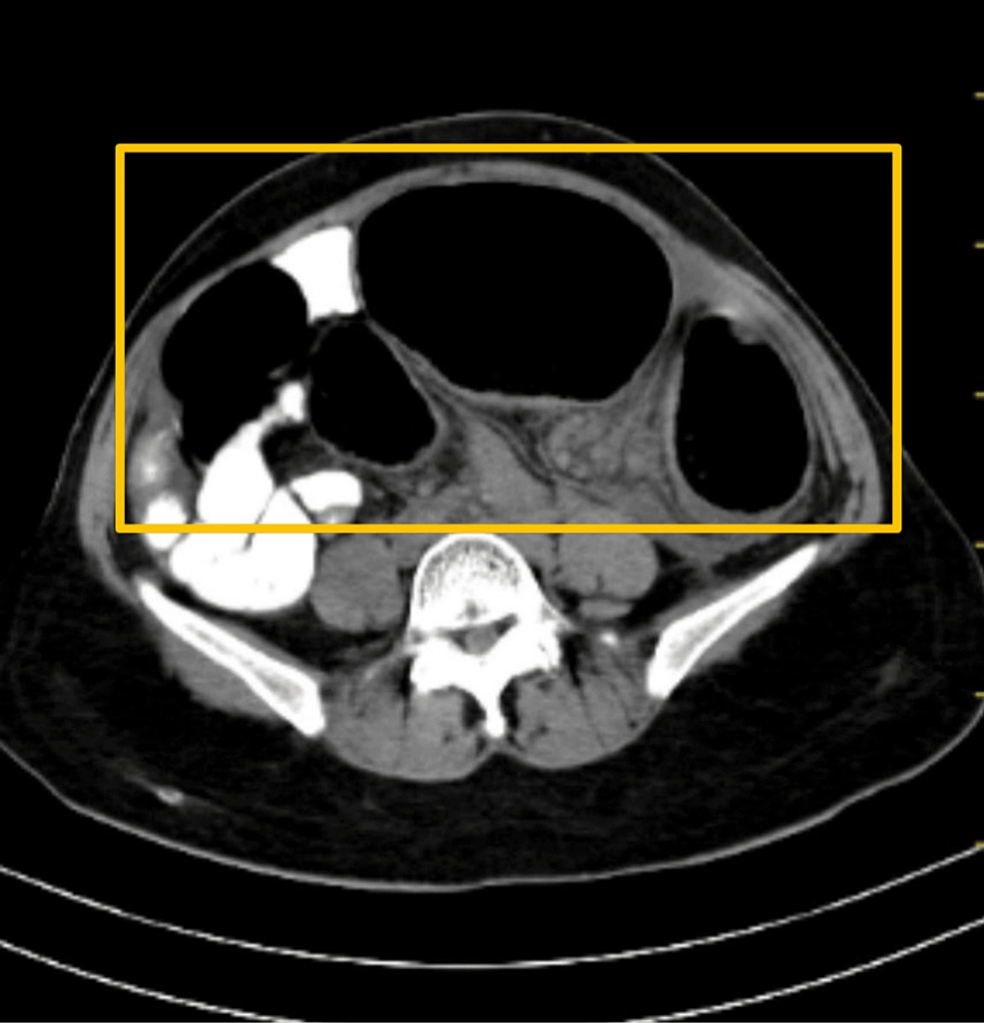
CT of the abdomen showing a dilated sigmoid loop, suggestive of acute intestinal obstruction
